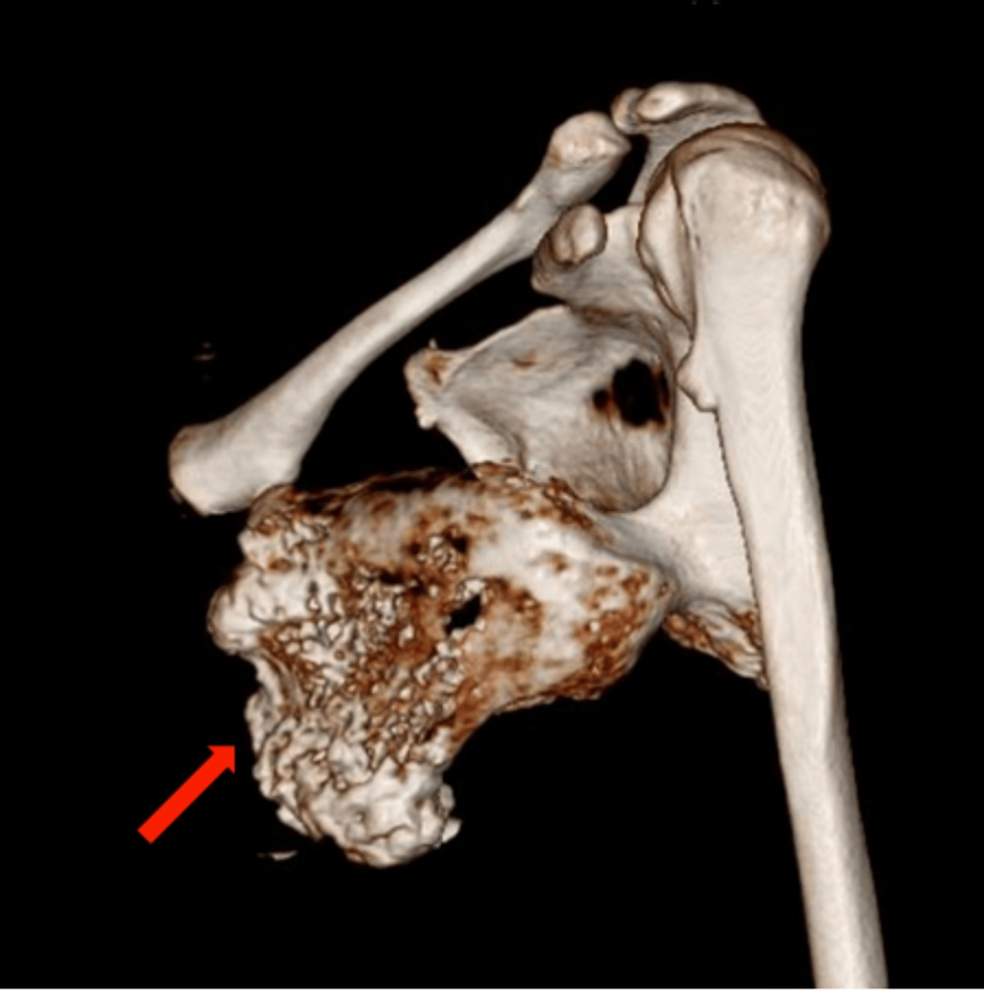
Three-dimensional CT scan showing the bony mass with the stalk attached to the scapula CT: computed tomography
